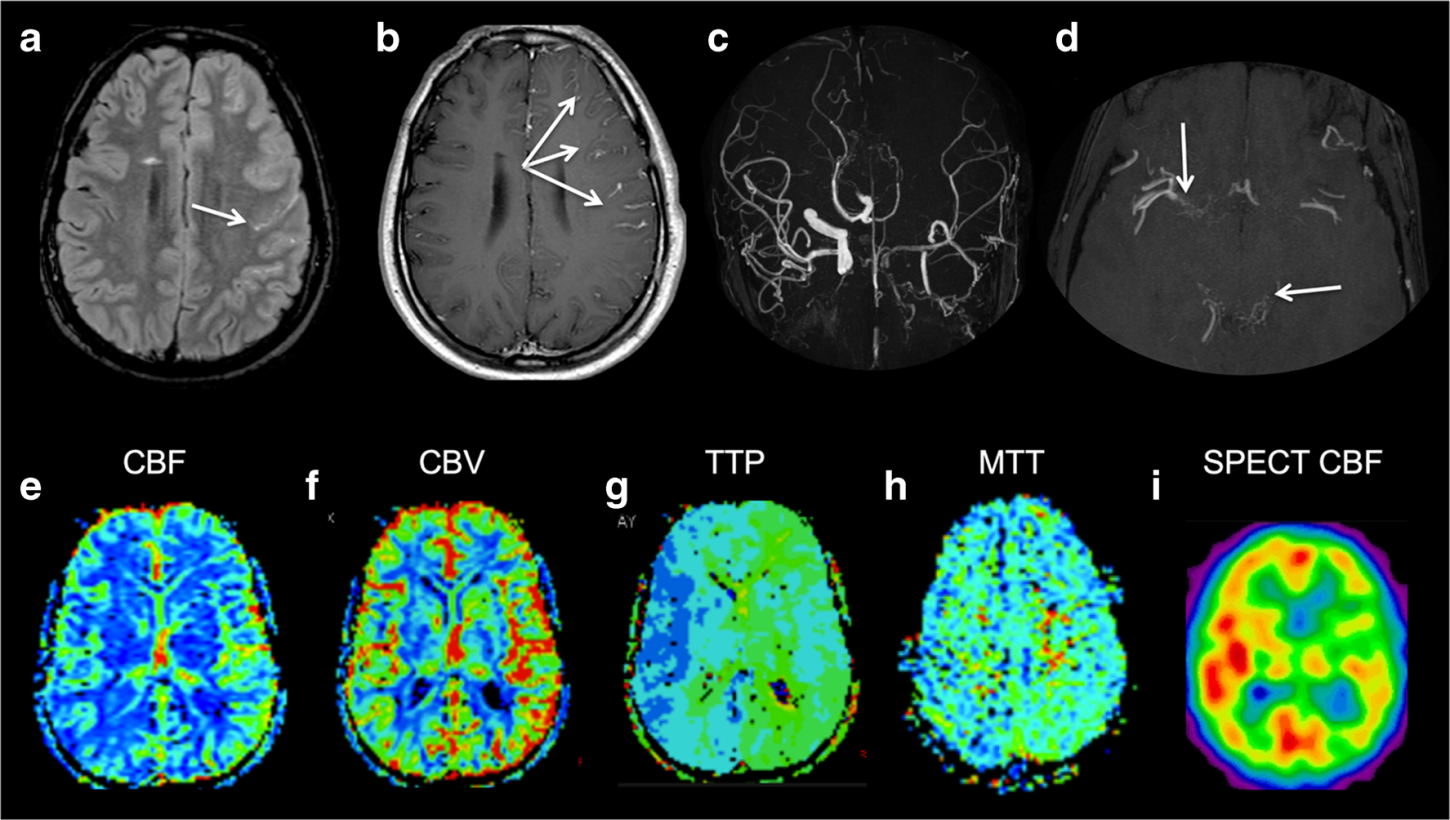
Imaging findings in patient #1. FLAIR image (a) shows “ivy sign” in the subarachnoid spaces of the left cerebral hemisphere (arrows) which corresponds to linear enhancement on contrast enhanced T1-weighted sequence (b). MR angiography maximum intensity projection (c) showing left ICA occlusion (related to the presence of a trigeminal neurofibroma infiltrating the cavernous sinus) and multiple MM stenoses on the right distal ICA, A1 segments, and left P3 and P4 segments. Multiple thin collaterals were observed in the right sylvian fissure and in the quadrigeminal cistern (arrows on d). On DSC-PWI, CBF (e) is symmetric, while CBV (f) and TTP (g) maps show an increase in the left hemisphere. TTP (g), with an increase in MTT (H) at the level of the left parietal lobe. Finally, SPECT demonstrates left cerebral hemisphere hypoperfusion (I)
